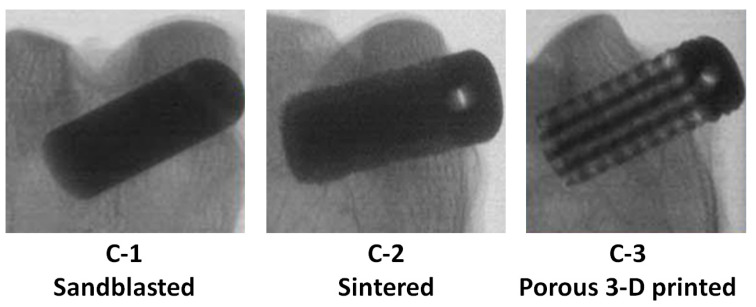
Implanted sample plain X-ray image. On the left image is C-1, the center C-2, and on the right side, C-3.
